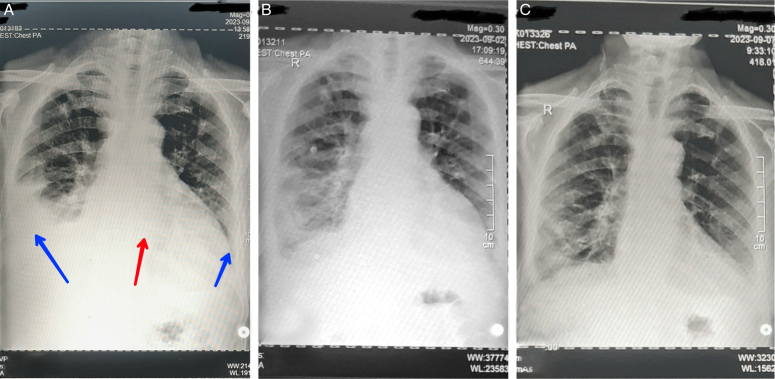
Chest X-ray. (A) Pleural effusion in both lungs, with a more pronounced loss of the costo-phrenic angle in the right lung (blue arrow). Additionally, there is pericardial effusion, indicated by a spherical enlargement of the cardiac shadow, resembling a water bottle (red arrow). (B) The day after pleural aspiration. (C) One week after pleural and pericardial fluid aspiration.
